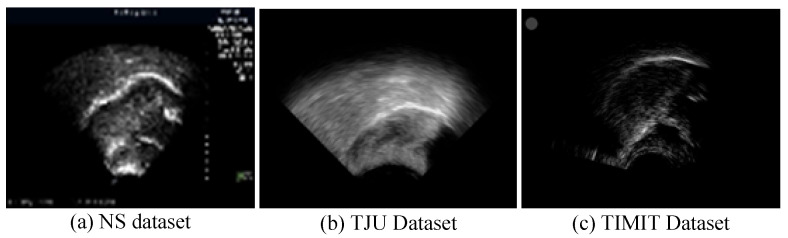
Imaging results of the ultrasonic transducer, with (a–c) representing samples from the NS dataset, TJU dataset, and TIMIT dataset, respectively. The prominent line at the center represents the tongue’s lateral contour, thus extending from the tongue’s root on the left to its tip on the right.
